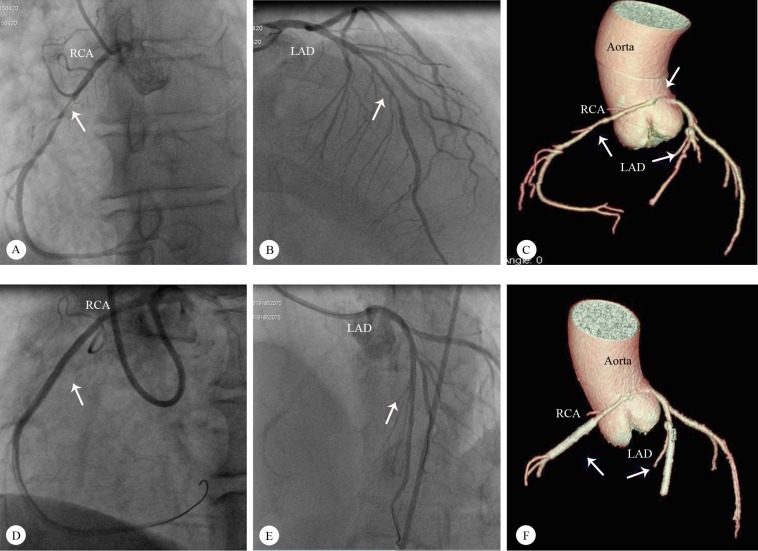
A) Left anterior oblique angiographic view of the RCA originating from the left sinus of Valsalva and a severe midportion RCA stenosis (arrow indicates the stenosis); B) Right anterior oblique cranial angiographic view of a severe midportion LAD stenosis (arrow indicates the stenosis); C) Computed tomographic left anterior angiographic imaging of the course of the RCA originating from the left sinus of Valsalva (arrow indicates the RCA origin and stenoses in the RCA and the LAD); D) Left anterior oblique angiographic view of the successful percutaneous intervention on the midportion of the RCA (arrow indicates the stent); E) Left anterior oblique cranial angiographic view of the successful percutaneous intervention on the LAD (arrow indicates the stent); F) Computed tomographic left anterior angiographic imaging of the stents in the LAD and the RCA 1 month after percutaneous intervention (arrow indicates the stent)
